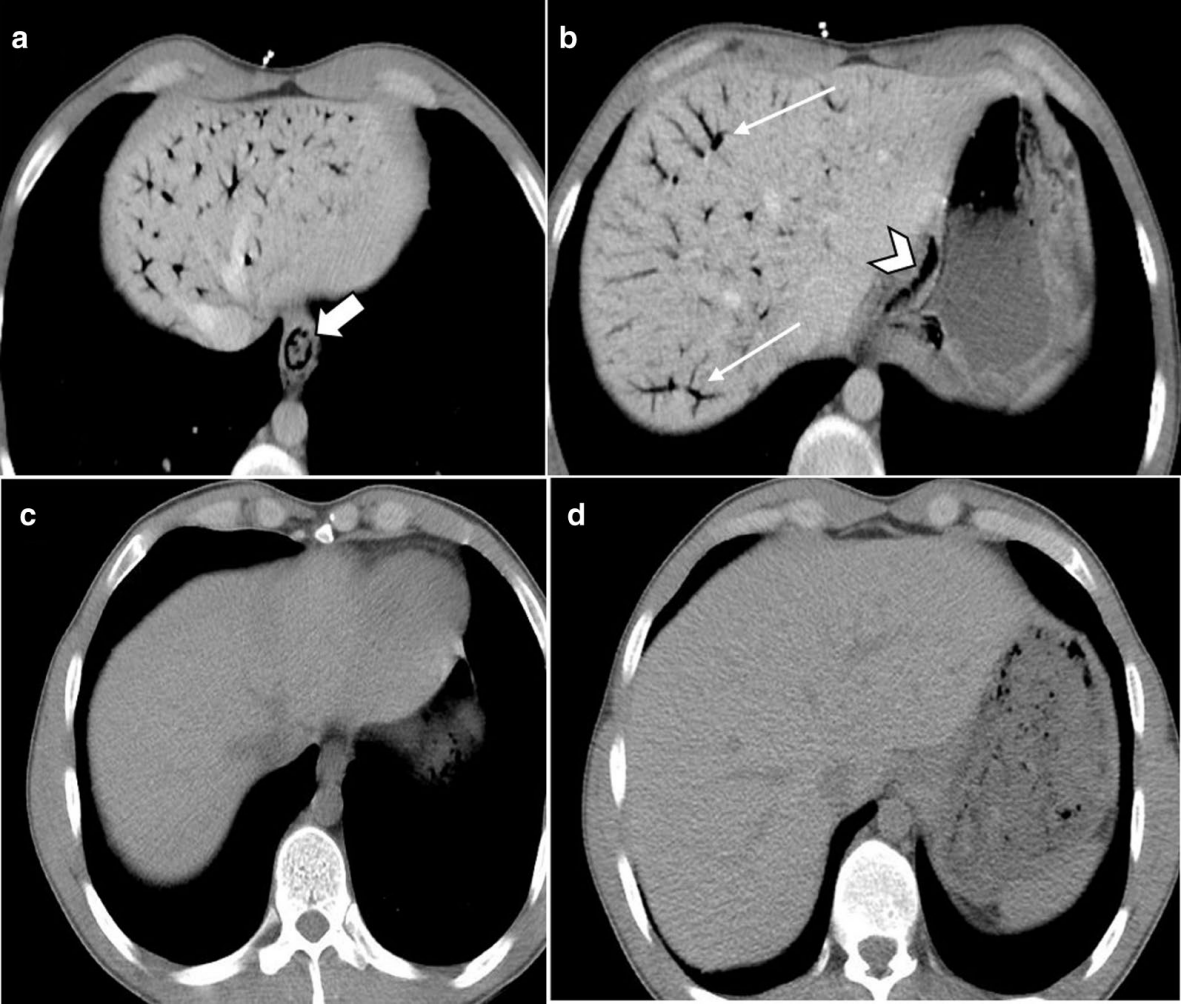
A 23 y/o male presented following hydrogen peroxide ingestion in suicide attempt, found to have transient gastro-esophageal pneumatosis and portal venous gas secondary to esophagogastritis. a, b Initial contrast-enhanced CT demonstrates intramural air within the distal esophagus (thick arrow) and stomach (arrowhead). There is associated extensive portal venous gas (thin arrows). c, d Follow-up CT demonstrates interval resolution of portal venous gas, esophageal and gastric pneumatosis. Patient was treated conservatively as there was no evidence of perforation on upper endoscopy
